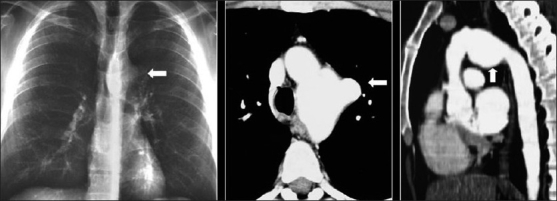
This human immunodeficiency virus patient suffered from gram negative septicemia, which was successfully treated. However, routine physical examination revealed an audible bruit on thoracic auscultation. The chest X-ray shows a prominent hump over the proximal descending aorta due to mycotic aortic aneurysm. The axial computed tomography (CT) and coronal CT reconstruction elegantly demonstrate the abnormality
